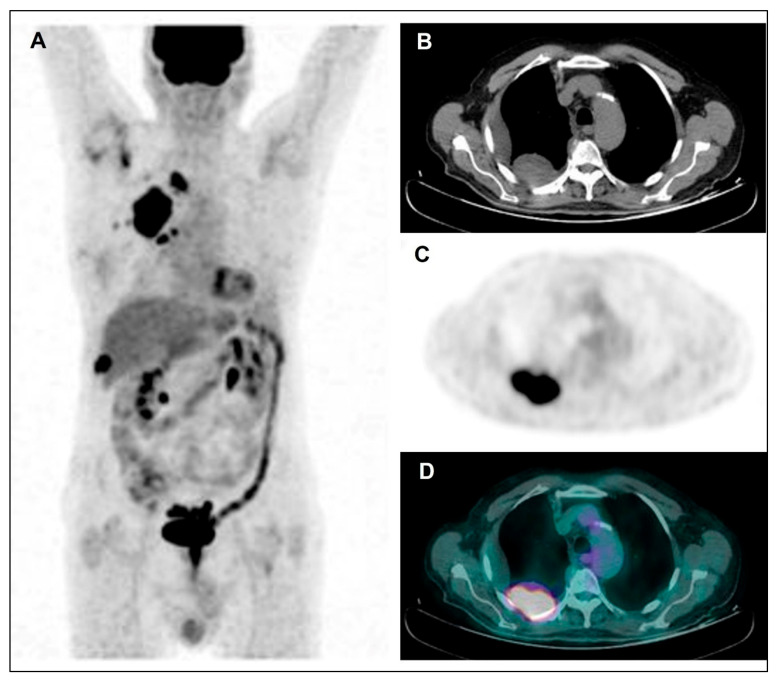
Maximum Intensity Projection—MIP (A), coregistered low-dose CT (B), ^18^F-FDG PET (C), and fused PET/CT (D). Images in a male patient with multiple right pleural thickenings showing high tracer uptake along with pleural effusion: the largest and most active lesion is located in the posterior side of costal pleura, as shown in the figure. Afterwards, the patient underwent VATS and pleural sampling with diagnosis of diffuse bi-phasic MPM.
